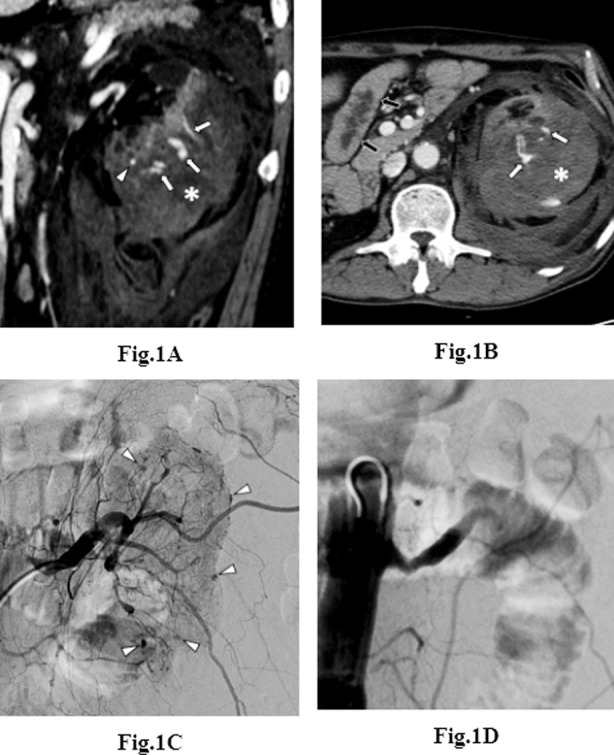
A 44-year-old man (no. 2) with diffuse cortical hemorrhage. a, b Coronal (a) and axial (b) contrast-enhanced computed tomography scans showing multiple foci of contrast extravasation (arrows) and pseudoaneurysm (arrowhead) along the periphery of the renal cortex with a large subcapsular hematoma (asterisks). Note the concentric jejunal wall thickening indicating an ischemic change (black arrows in b). c Left renal angiogram showing multiple pseudoaneurysms (arrowheads) along the periphery of the renal cortex. d Aortogram showing complete occlusion of the left renal artery after embolization with gelatin sponge particles and polyvinyl alcohol.
